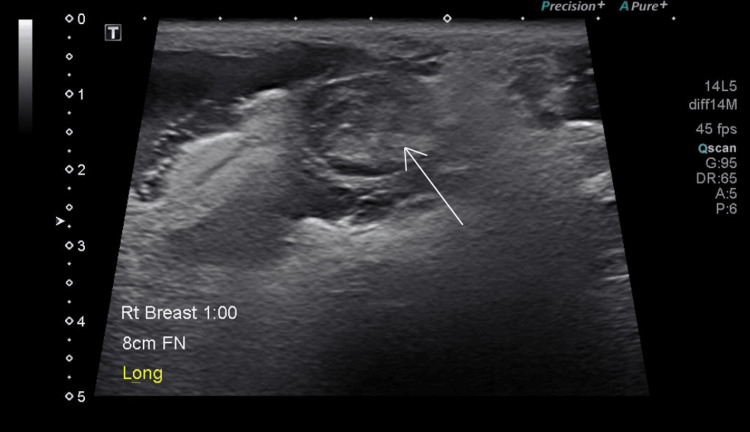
Single gray-scale ultrasound demonstrating thrombosed pseudoaneurysm There is surrounding hematoma and blood products.
